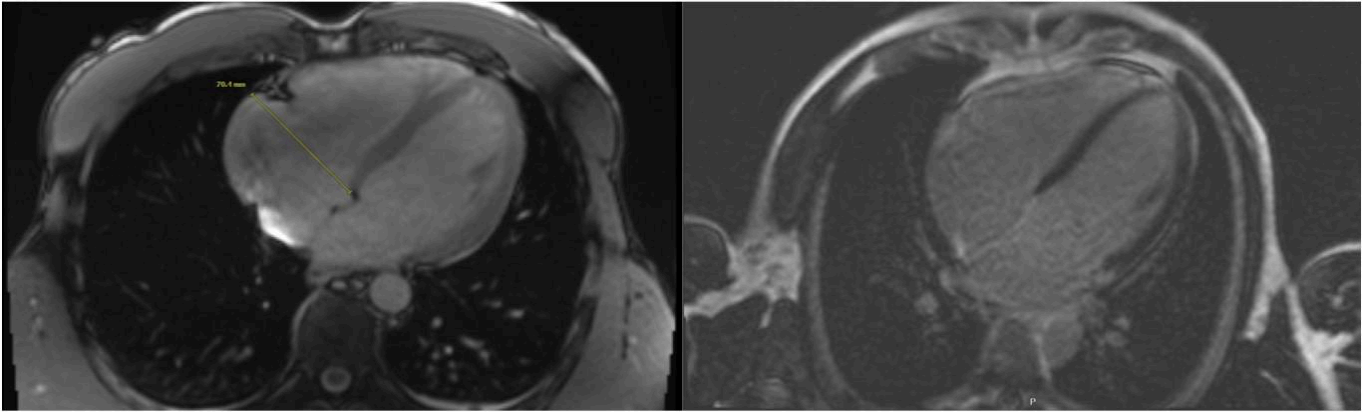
The cardiac magnetic resonance imaging shows: (left) still frame of an axial SSFP cine sequence, demonstrating a dilated right ventricle measuring around 70 mm at the base (volume index of 92 cc/m^2^) and without focal wall motion abnormalities to suggest arrhythmogenic right ventricular cardiomyopathy; (Right) Late gadolinium enhancement sequence, showing no signs of myocardial fibrosis.
